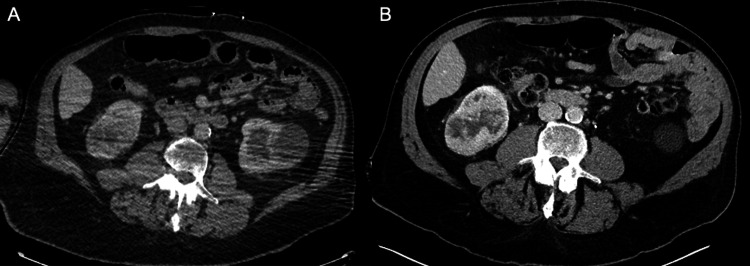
Case 2 - imaging of the right renal mass prior to radiation therapy. (A) Axial computed tomography (CT) of the chest, abdomen, and pelvis demonstrates a mixed solid and cystic mass at the lower pole of the right kidney. (B) Follow-up CT five months later shows interval enlargement of the mass.
